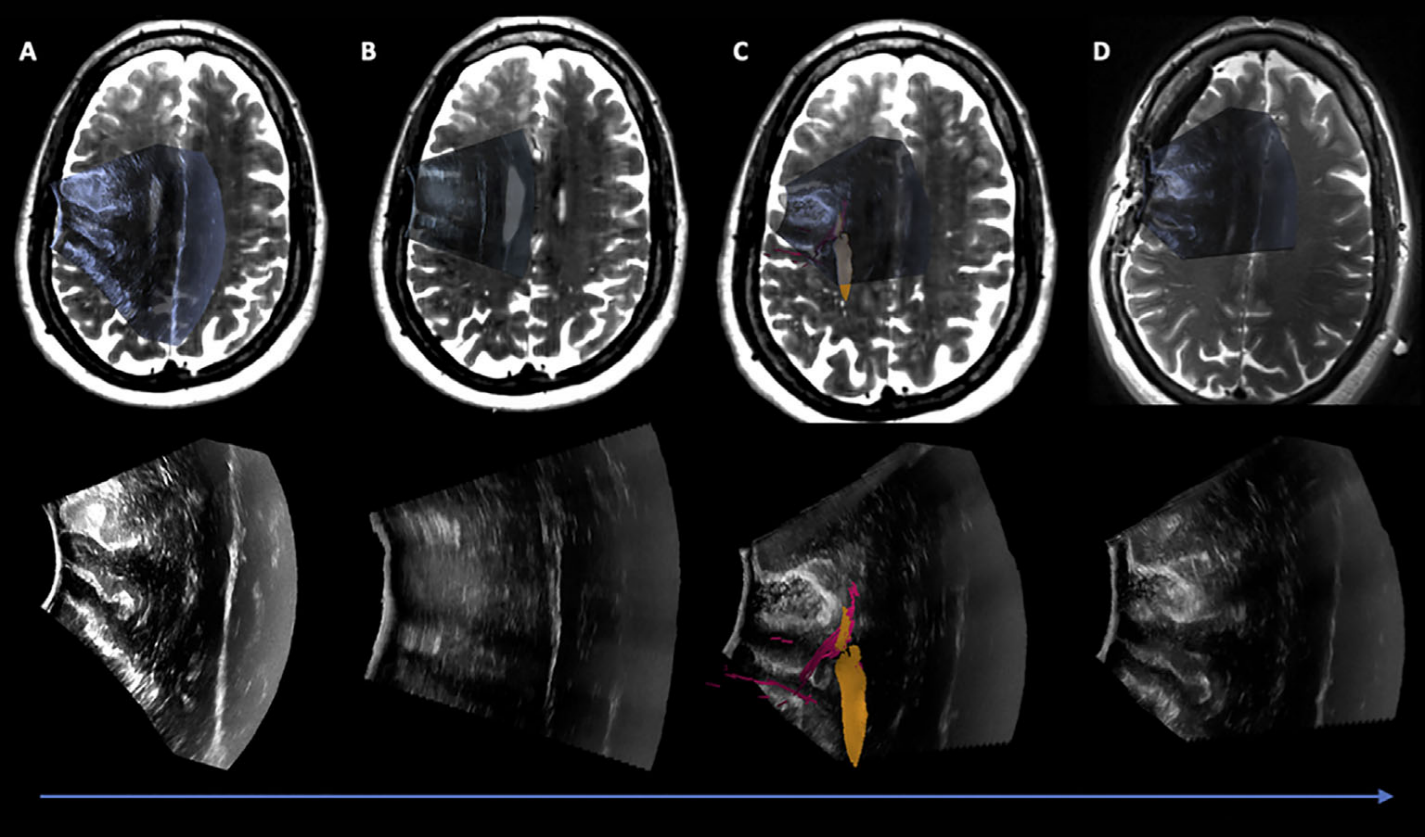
Case 1 Post hoc manually aligned preoperative axial T2-weighted imaging with (A) iUS-1, (B) iUS-2, (C) iUS-3 with right Corticospinal Tract (yellow) and Frontal Aslant Tract (red), and (D) iMRI axial T2-weighted imaging manually aligned with iUS-3. On D it is possible to observe a gross total resection on iUS and confirmed by iMRI.
